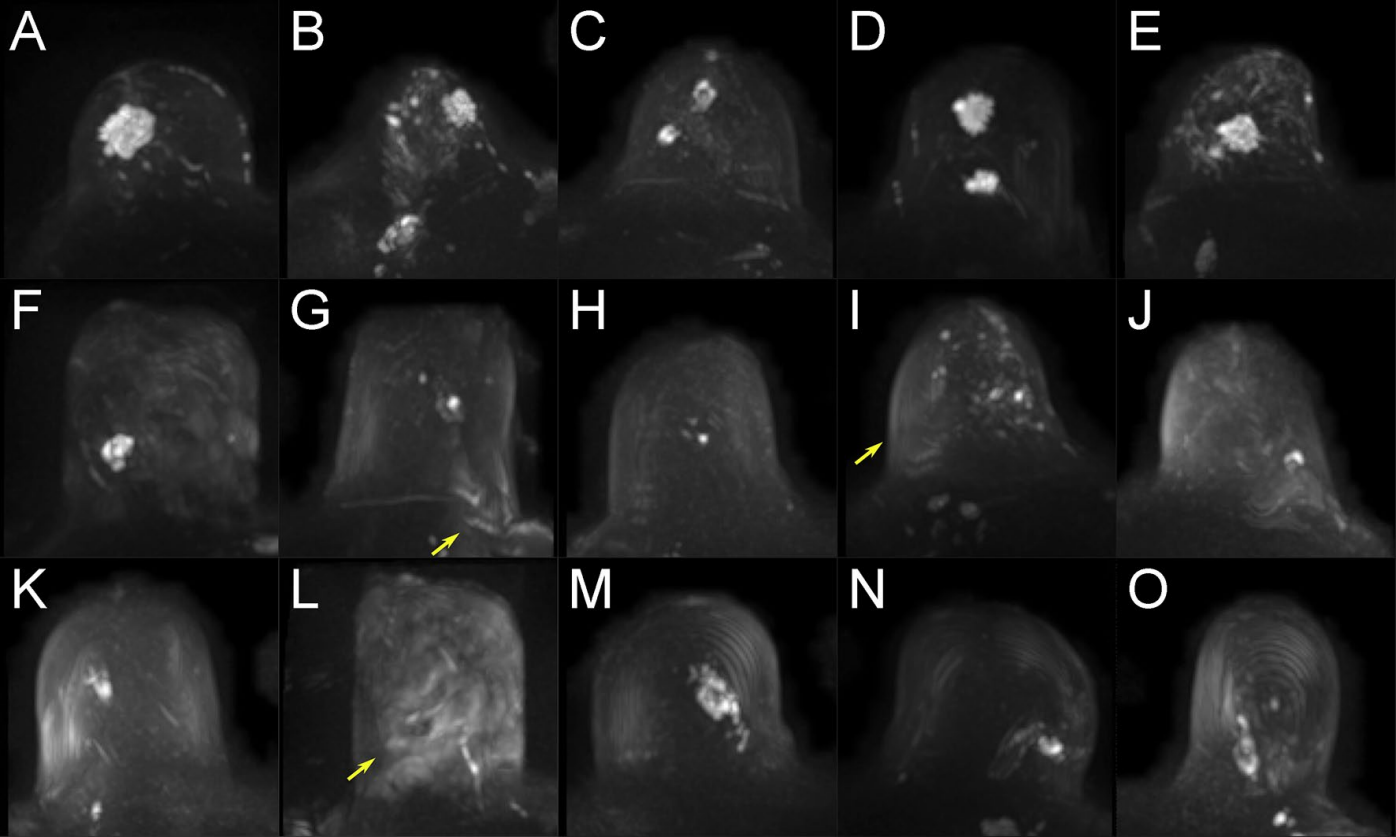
BI-RADS 6 lesions in clinical cases (examples). Each tile of the figure presents the left or right breast of one clinical case with a diagnosed BI-RADS 6 lesion. Row 1 (A–E) shows images without the presence of artifacts. Row 2 (F–J) shows images that contain artifacts with no or moderate influence on the diagnostic assessment. Row 3 (K–O) shows images with artifacts that significantly impede the diagnostic evaluation. Artifacts in DWI often originate from multiple technical and/or patient-related sources that may be interdepend and thus it is not always possible to attribute one specific artifact source. The arrows mark regions of artifacts within the images with possible contributing factors related to distortion (e.g. visible in G), insufficient fat suppression (e.g. visible in I, L), related to remaining surface coil flare (e.g. also visible in I), and pulsation related signal drops in DWI (e.g. also visible in L).
